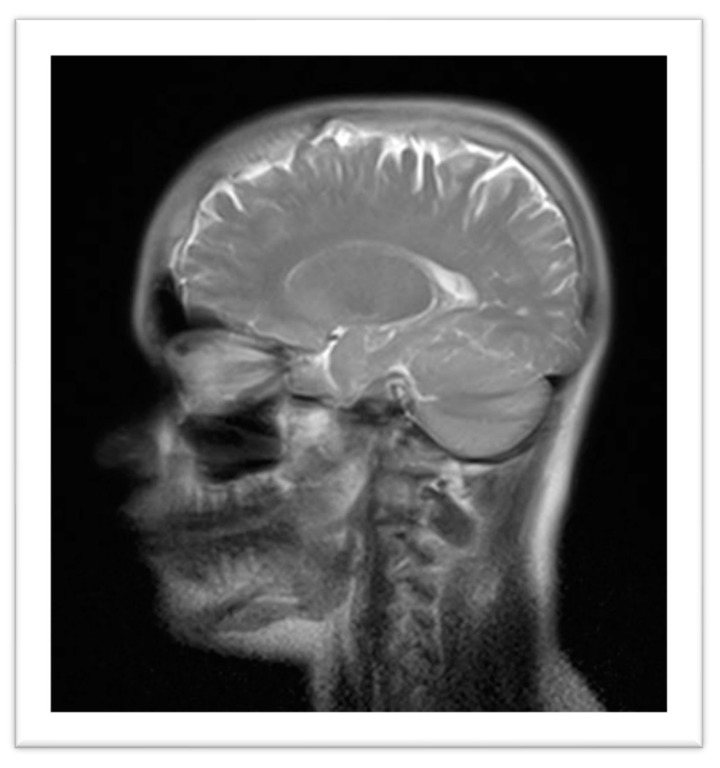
Brain Magnetic Resonance Imaging: calcification in the anterior aspect of the falx cerebri, hyperostosis of the inner calvaria table in the frontotemporoparietal regions, and asymmetry of the petrous apices.
